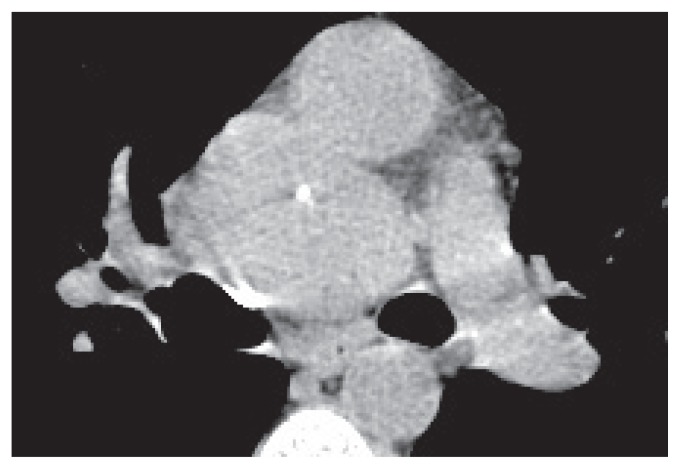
Unenhanced axial CT image demonstrating the Castleman nodal mass abutting the left main pulmonary artery and ascending aorta. A central spot of calcification is present.
